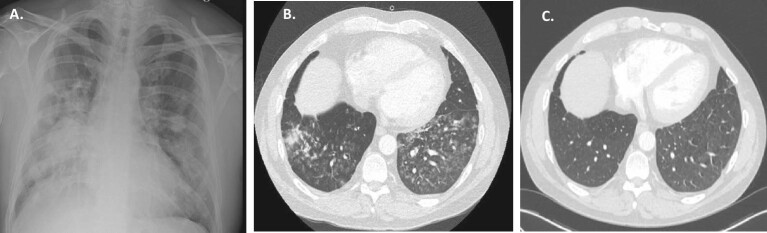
(A) Patient 7 at presentation: chest X-ray: bilateral consolidation; (B) Patient 7 at presentation: chest CT scan: diffuse ground-glass opacity of the lungs; (C) Patient 7 after 6 months: chest CT scan: significant improvement.
